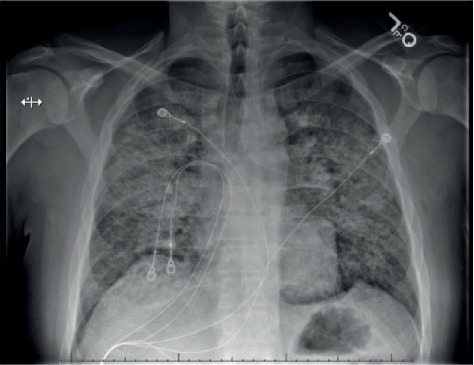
Chest X-ray showing extensive patchy bilateral consolidation.
